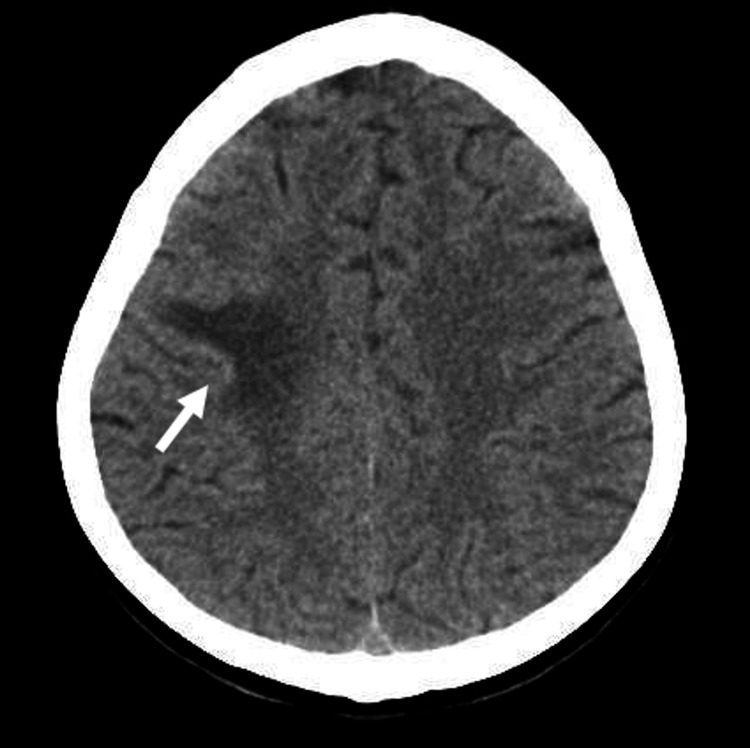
CECT demonstrates predominantly right posterior frontal subcortical hypodensity. CECT: cranioencephalic computed tomography
